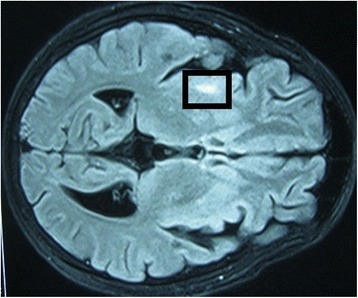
Magnetic resonance image: transverse section of the brain on the third day of admission. This section shows high T2 signals in the right hippocampal gyrus, right putamen, and the head of the caudate nucleus, suggestive of infarctions
